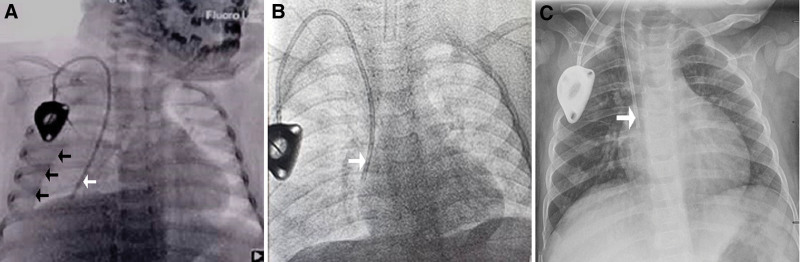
Intraoperative and postoperative images of the second catheterization. (A) Fluoroscopy shows fluid accumulation (black arrows) in the right pleural space with passive atelectasis. The tip of the catheter (white arrow) was in the right pleural space. (B) Fluoroscopy shows the tip of the catheter (white arrow) in the right atrium. (C) Chest roentgenogram shows the tip of the catheter (white arrow) in the right atrium.
